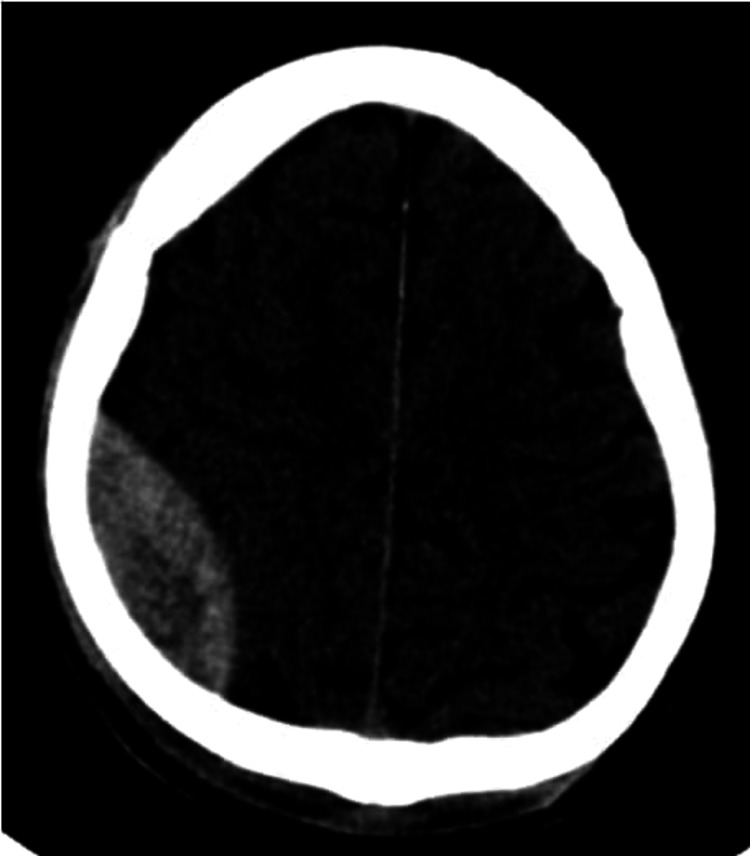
Axial NECT shows an extradural hemorrhage in the right parietal region NECT: non-contrast-enhanced computed tomography
